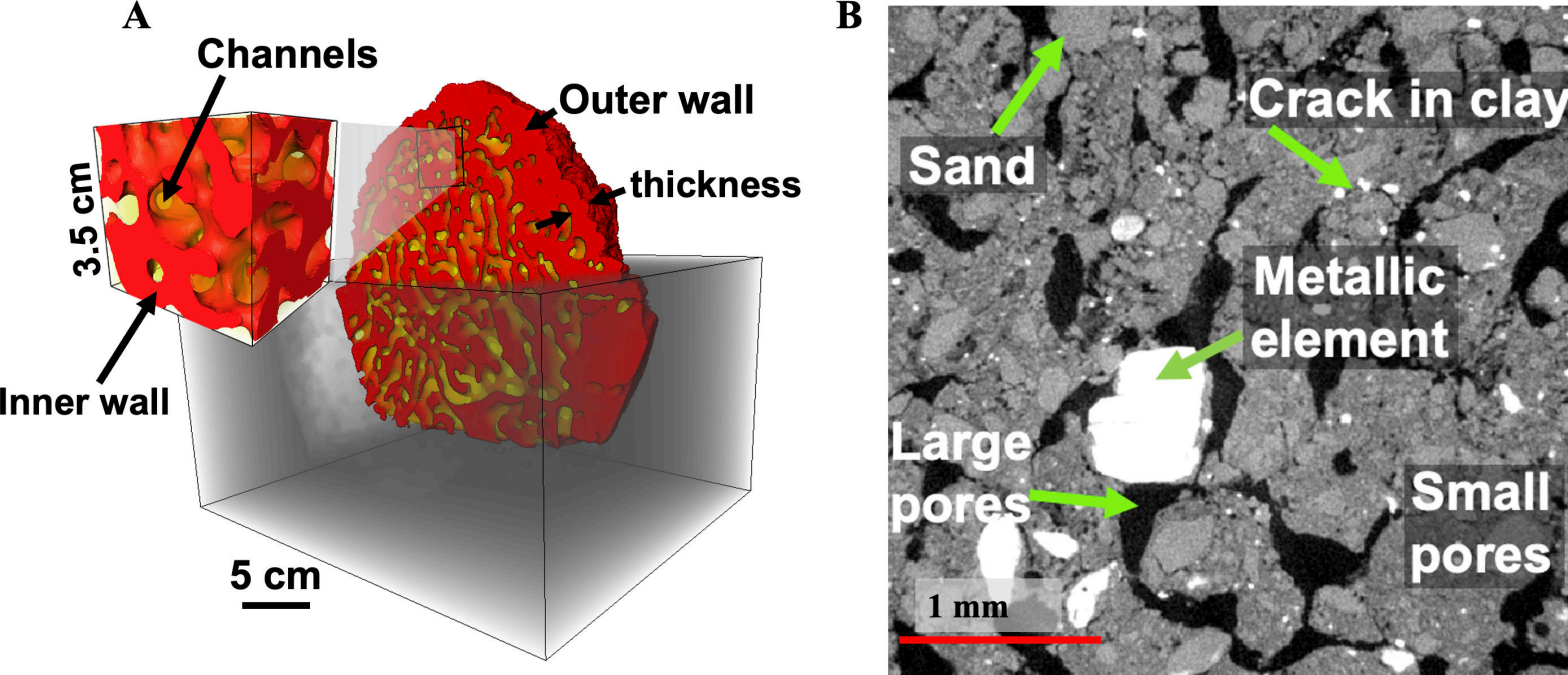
X-ray tomography of a termite mound from the *Trinervitermes geminatus* species. (A) Three-dimensional image showing channels, inner walls and outer walls and (B) a vertical cross-section of an X-ray micro-tomography scan showing micropores of different sizes, metallic elements sand and cracks (adapted from Singh *et al.* [50]).
